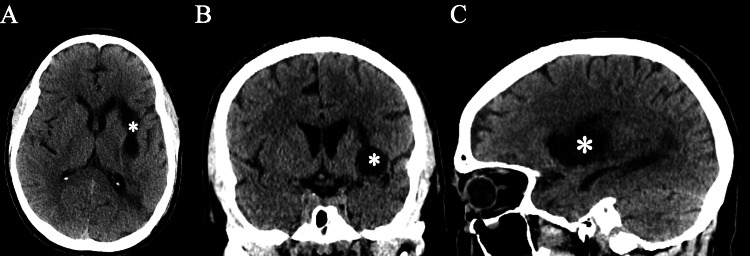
CT of the brain - axial (A), coronal (B), and sagittal (C) cuts approximately two months post-operation demonstrating encephalomalacia (*) of the basal ganglia, involving the globus pallidus and the internal capsule complex
